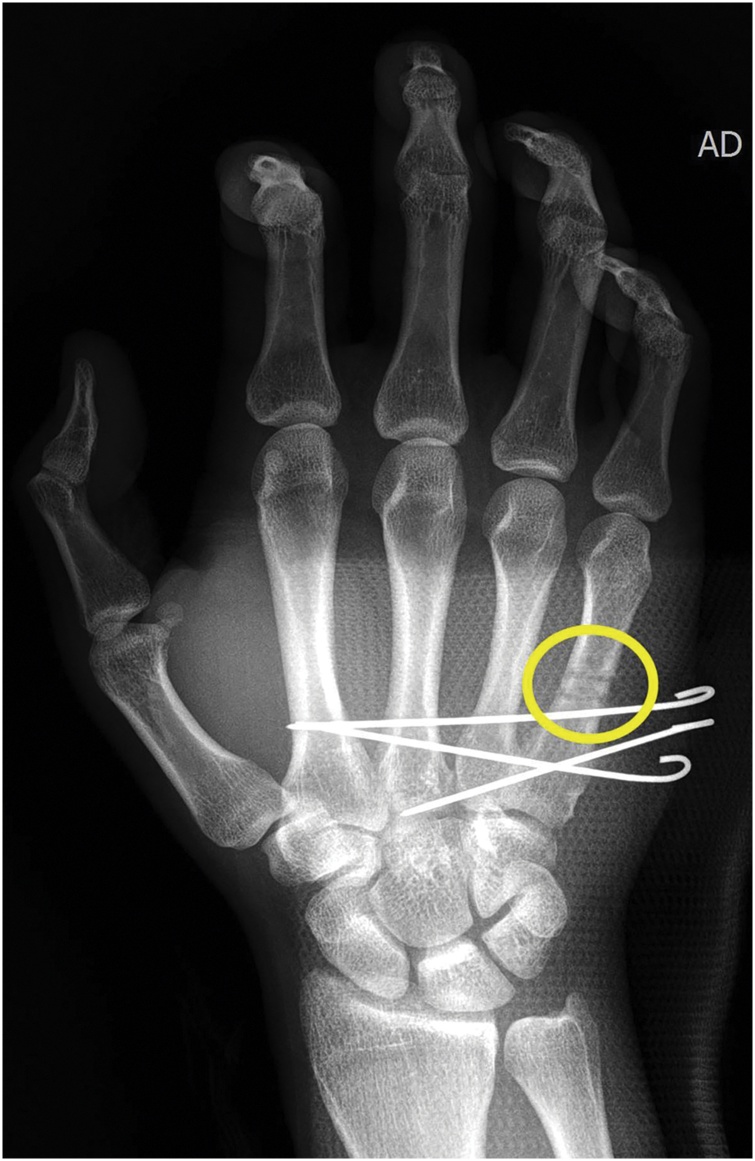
Intra-operative, immediate post-operative X-ray. Although closed reduction was attempted, the CMC joints were unstable. Following our protocol, reduction was obtained by longitudinal traction and lateral pressure on the displaced bone and fixation with a transarticular pin and transfixation pins into the adjacent metacarpal was planned. During these procedures, the resident of our team made several attempts to insert transfixation pins with 1.1 mm K-wire into the metacarpal shaft, but each attempt failed. After final fixation, immediate postoperative X-ray showed the K-wire tracks (Yellow Circle).
